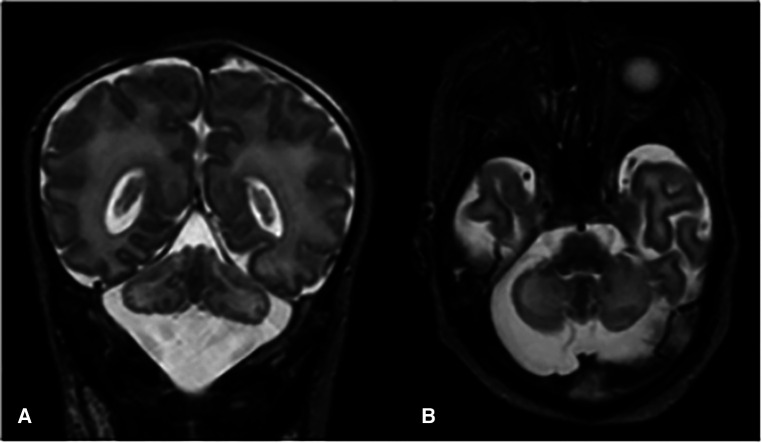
Coronal (A) and axial (B) T2-weighted images show global cerebellar hypoplasia with symmetric involvement of both hemispheres and vermis, along with a very large cisterna magna. There was no obvious abnormality of the brainstem. The cerebellar folia and fourth ventricle were not dilated, indicating there was no volume loss. The cerebellar foliation pattern was normal and there were no heterotopic nodules of gray matter, indicating there was no dysplasia.
